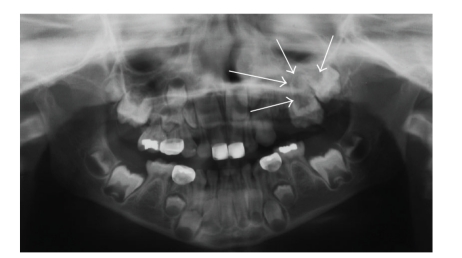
Panoramic radiograph showing lack of/delayed eruption of teeth in the left maxilla.
